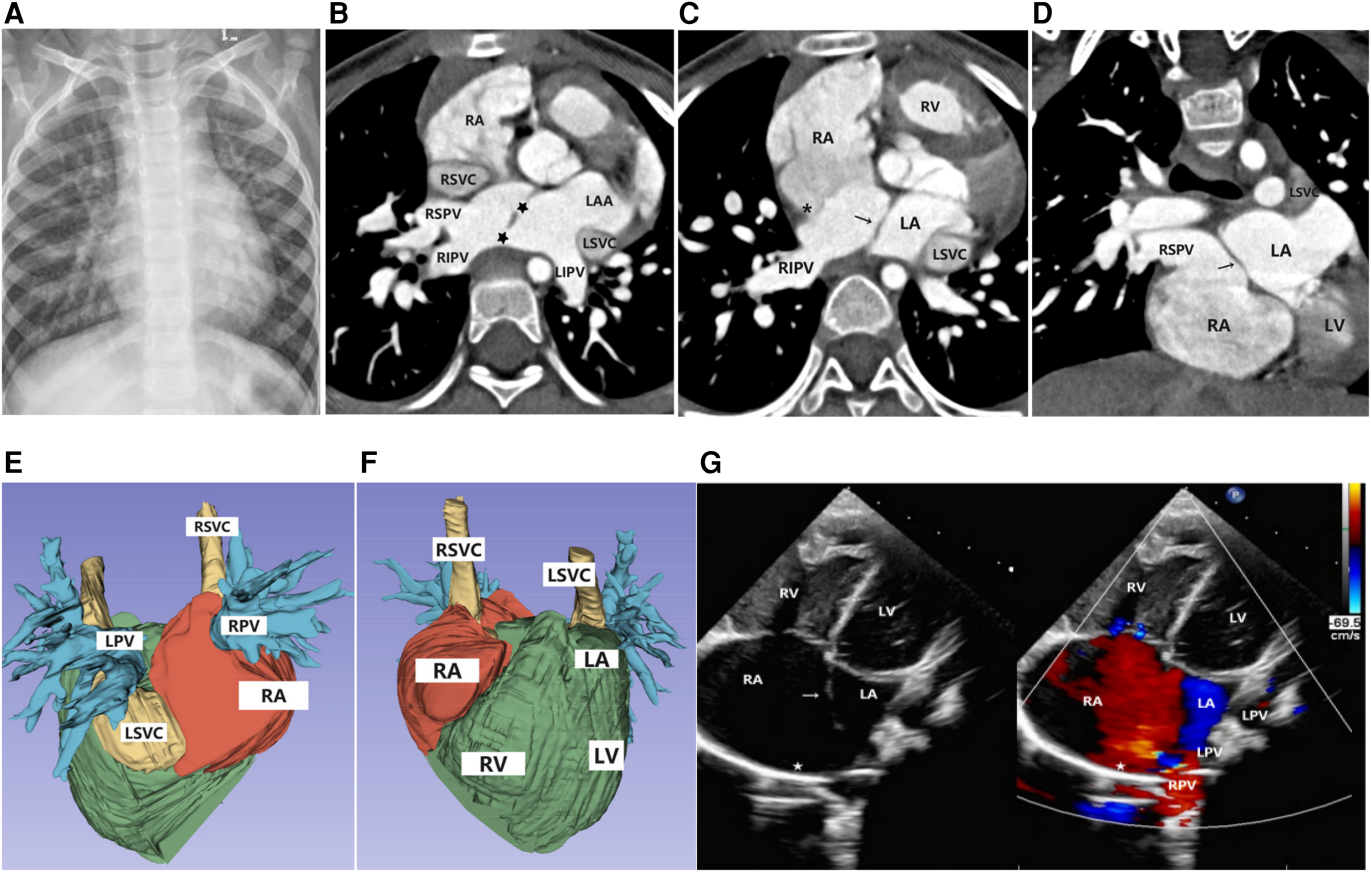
Imaging results for Case 1. (A) Chest x-ray: The cardiac silhouette was full, with increased and blurred pulmonary vascular markings in both lungs. (B–D) Axial and coronal view of CTA: The superior limbic band of the septum secundum is absent (*), the left superior and inferior pulmonary veins drain into the left atrium, and the right superior and inferior pulmonary veins drain into the anatomical right atrium (accessory atrium). A localized defect (
